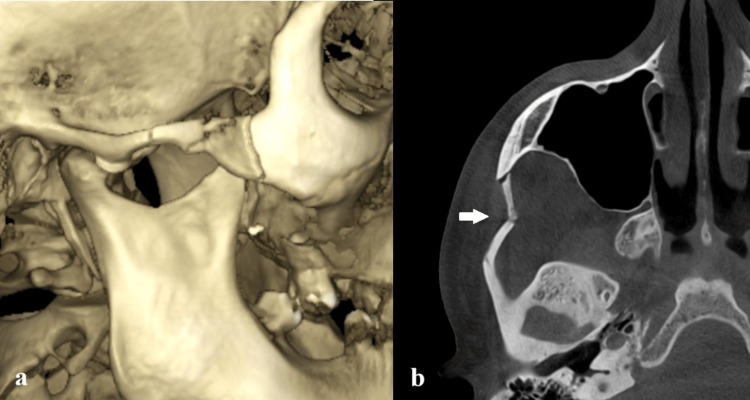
Preoperative dental volumetric tomography images of the patient; (a) the 3D reconstruction of the isolated zygomatic arch fracture; (b) the cone beam computed tomography (CBCT) image revealed a favorable isolated zygomatic arch fracture. The characteristic M-shape of the fractured zygomatic arch can be observed from the axial section of the CBCT.
